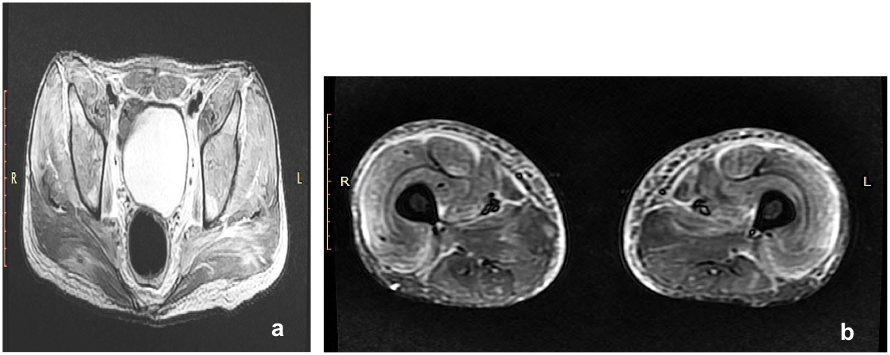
MRI of pelvis and thigh shows bilateral diffuse oedema in the muscle of the bilateral gluteal region and thigh suggestive of dermatomyositis
